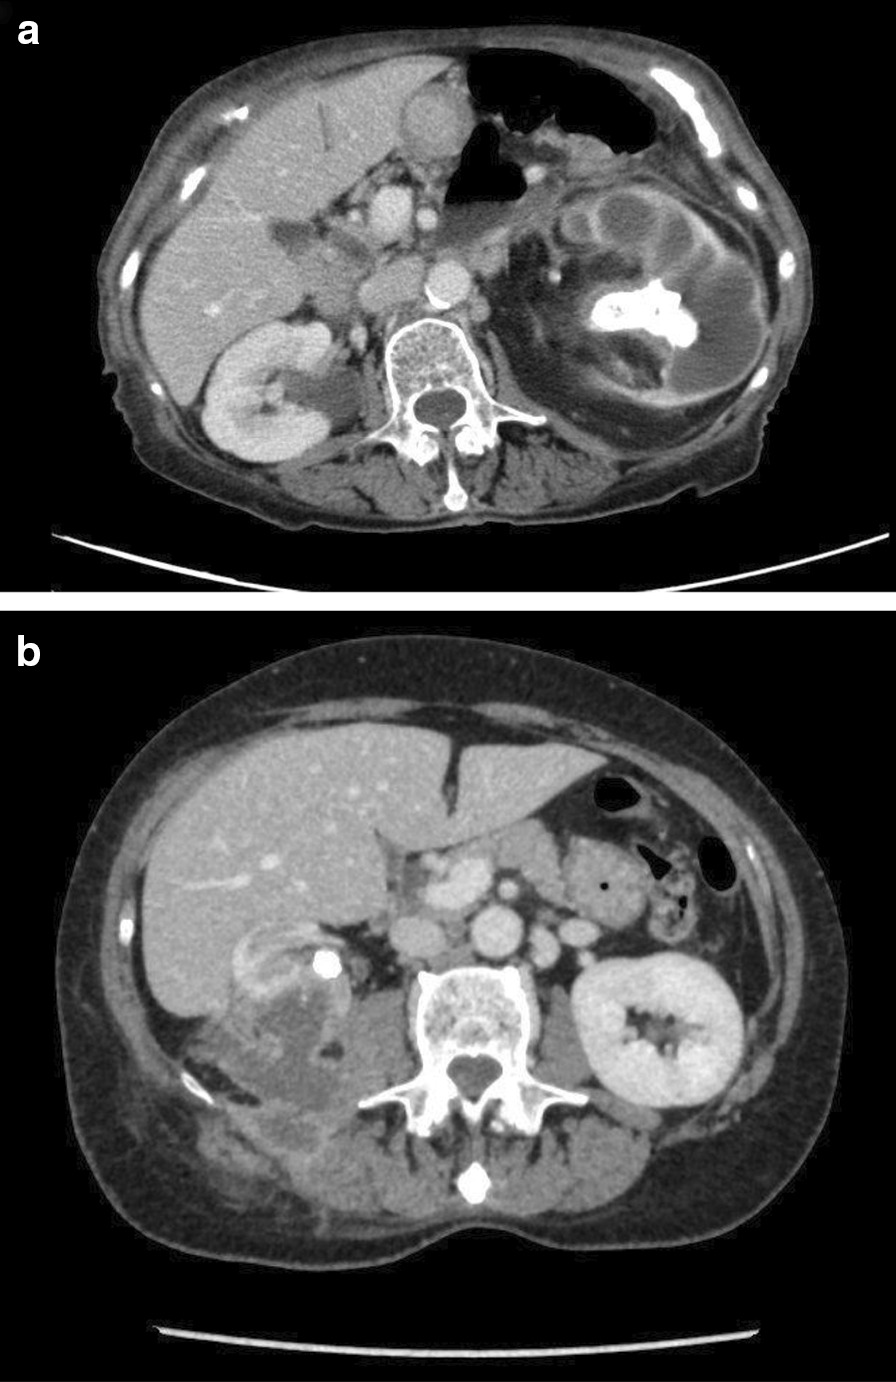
Axial CT scan images demonstrate obstructing left pelvic staghorn stone with associated grade IV hydronephrosis (Malek I) (a) and retroperitoneal collections surrounding the right kidney with a renal pelvic calculus (Malek III) (b)
